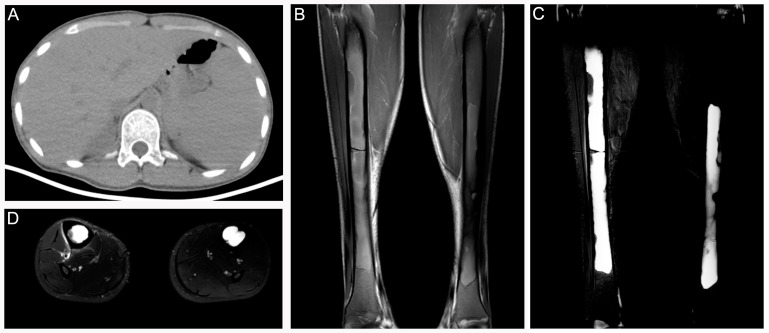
Patient 1: (A) Axial CT demonstrated marked hepatosplenomegaly. (B) Coronal T1 WI image following CT examination demonstrated low SI in the diaphyses of the femora extending to extremities. (C,D) There was high SI in these areas on coronal and axial STIR images with confined edges.
